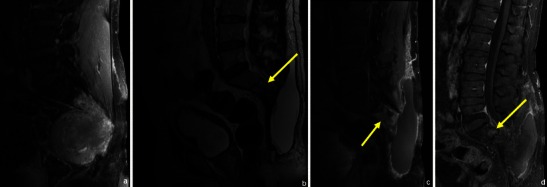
MRI applied in the diagnosis and follow-up of a patient with chordoma: this patient had a voluminous lesion destroying the distal part of the sacrum (a). He had radiotherapy and surgery and was re-evaluated 4 months later: MRI showed a posterior subcutaneous fluid collection and a localised area with high T2WI signal (arrow in b) and contrast enhancement (arrow in c) in the inferior spine, which could be due to either residual tumoral tissue or radiotherapy. Posterior follow-up however showed a localised round area of persistant enhancement, which proved to be local recurrent/residual tumour (arrow in d)
